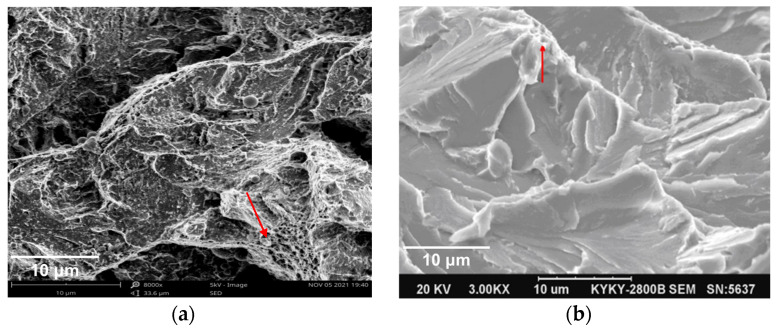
SEM images of the fracture morphology of (a) SA508 Gr.4N steel and (b) SA508 Gr.3 steel CT samples.
